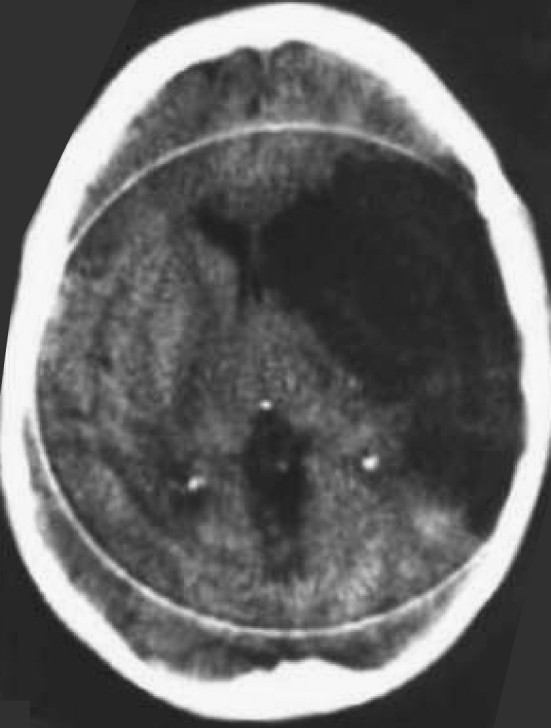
Transverse section from the cranial CT scan of JM. The darker area is consistent with infarction in the territory of the right middle cerebral artery. The lesion involves regions of the parietal, temporal, and frontal lobes (note that the bright outline circle is an artifact of the image production).
